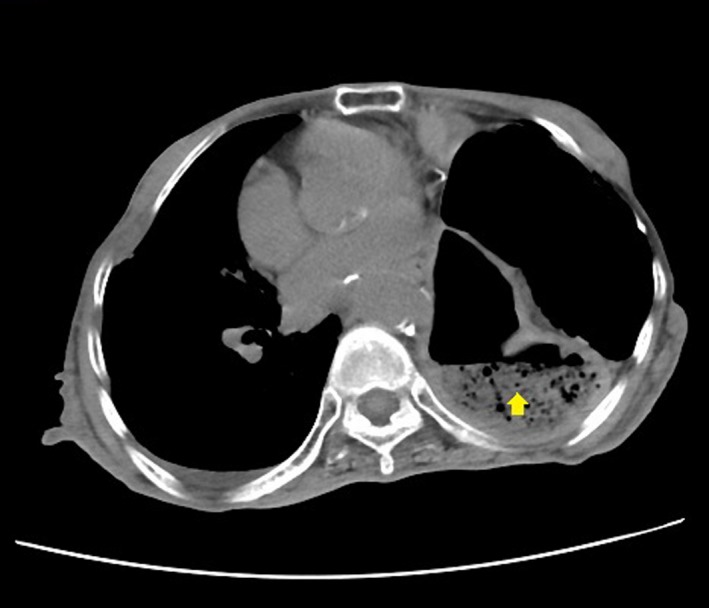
CT scan showing left diaphragm paralysis with the stomach (arrow) within the thoracic cage.
